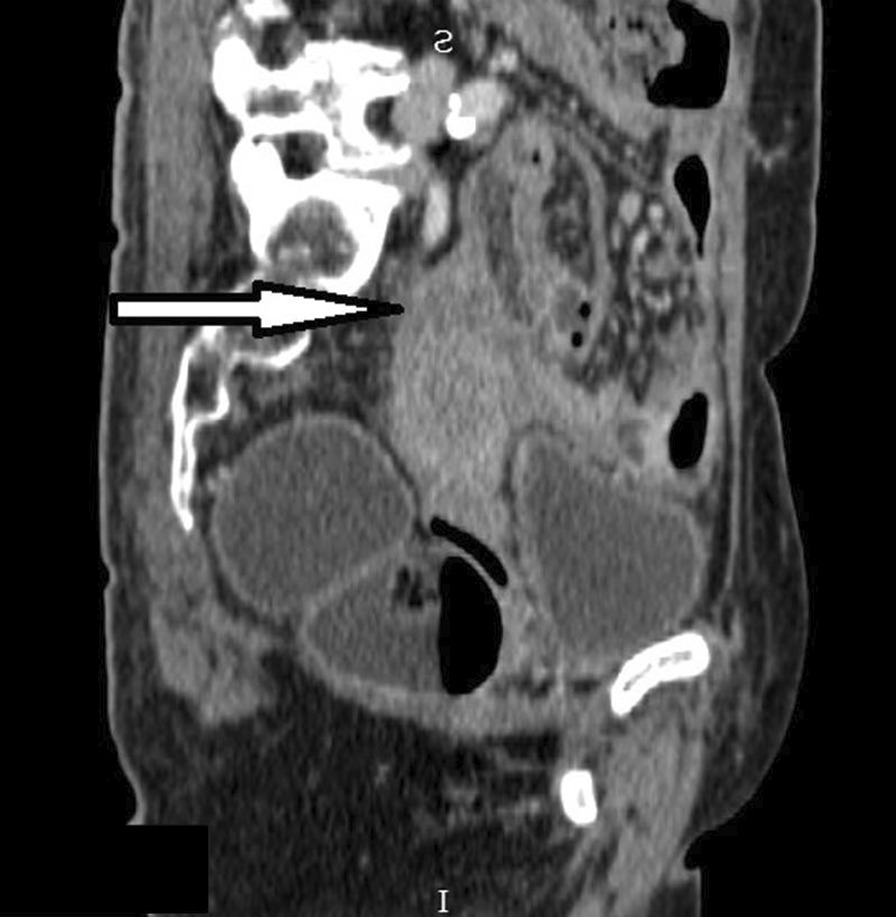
Sagittal computed tomography scan image demonstrating uterine fundus mass which has poor demarcation with the uterus inferiorly (arrow)
